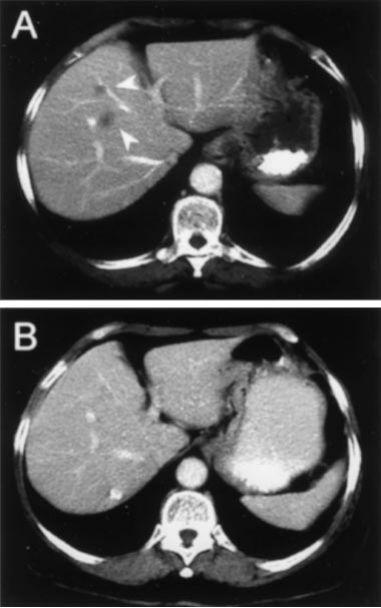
CT scan of the liver depicting metastases prior to therapy (A) and the CR after 4 cycles of intravenous fotemustine in combination with subcutaneous IL-2 and IFNα (B).
